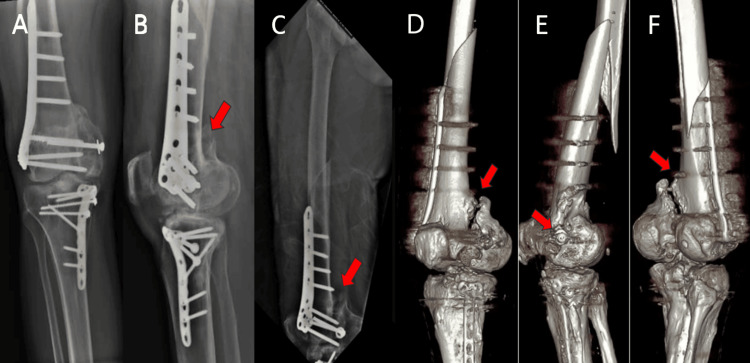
Preoperative plain radiograph and three-dimensional computed tomography (A) Plain radiograph of right knee (AP view); (B) Plain radiograph of right knee (lateral view); (C) Plain radiograph of right femur (lateral view); (D) 3D CT of right knee (posterior aspect); (E) 3D CT of right knee (medial aspect); (F) 3D CT of right knee (posterolateral aspect) The red arrow indicates the presence of a gap over the previous medial Hoffa fragment.
